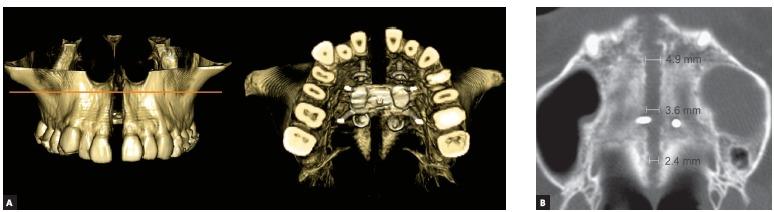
CBCT immediately after MARPE: A) frontal and occlusal views of tridimensional volumetric rendering, and B) axial slice with linear measurements of anterior, intermediate and posterior midpalatal widths.
